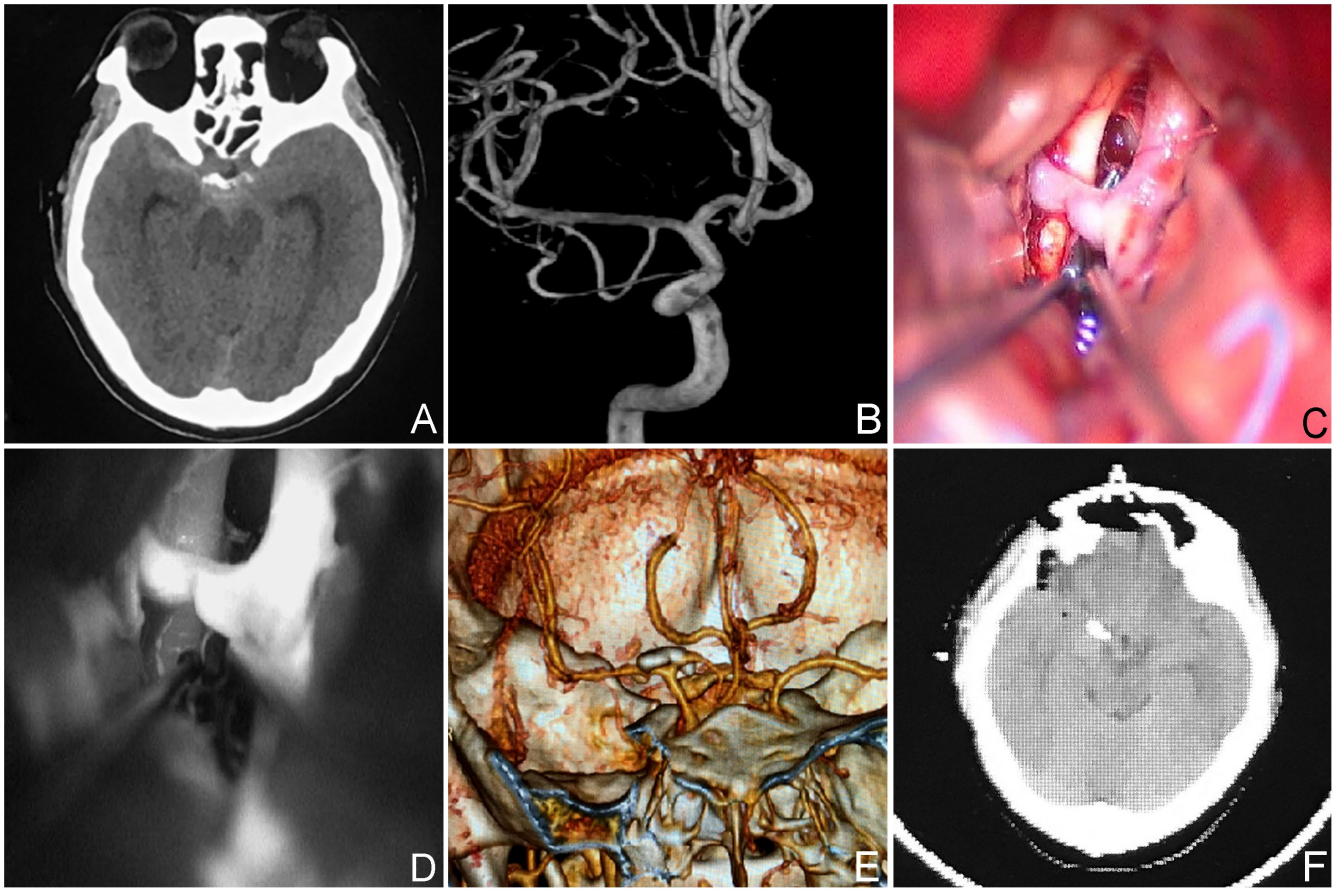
The neck of the aneurysm is situated within the inferior wall of the parent vessel (Type-IV). (A,B) A 43-year-old male presented with SAH on a CT scan indicating a ruptured aneurysm. The aneurysm originated from the inferior wall of the parent vessel, as determined by the DSA. (C,D) Intraoperative situation. Postoperative CTA (E,F) and CT scan.
